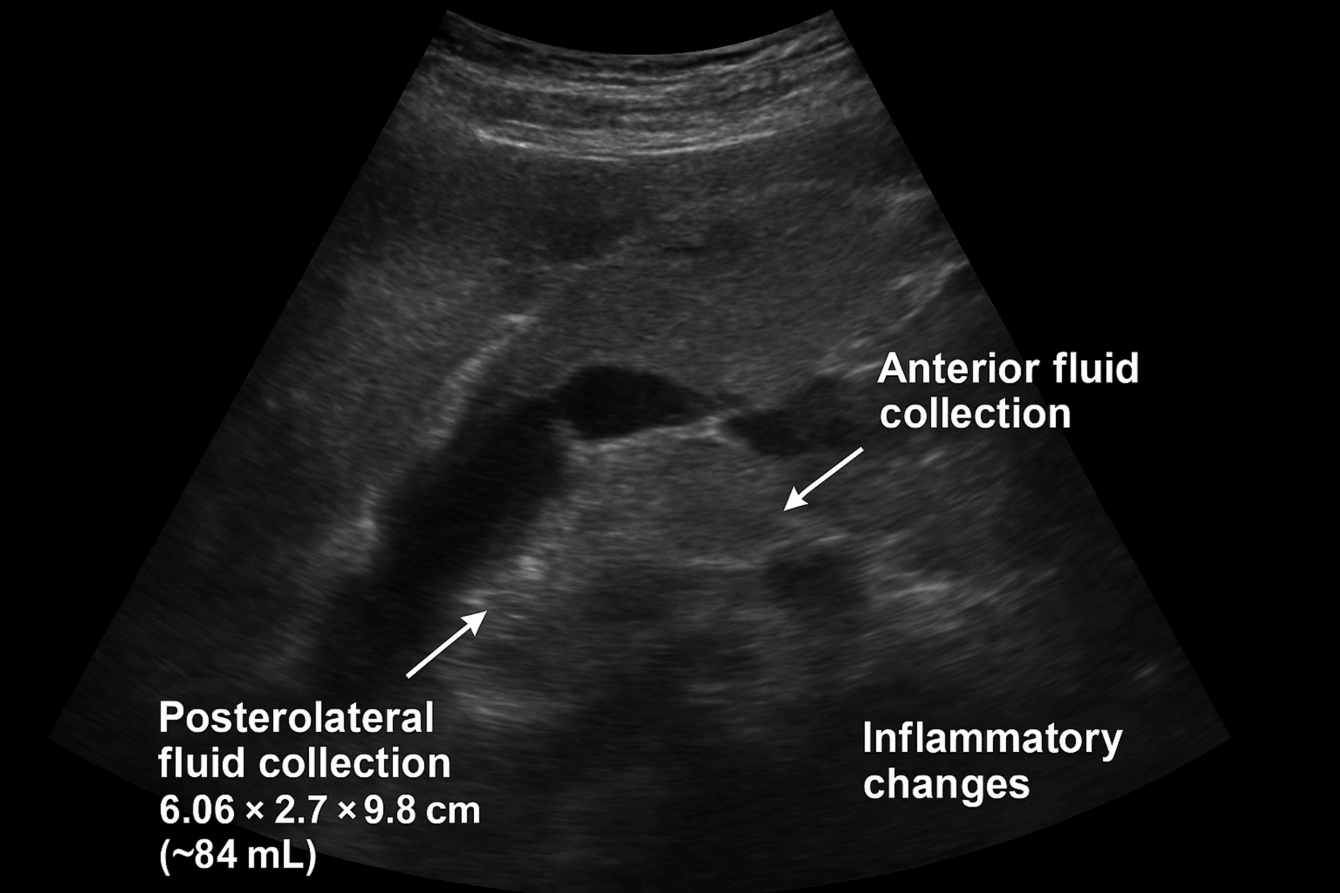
Abdominal ultrasound showing inflammatory changes in pancreatic parenchyma with fluid collections (Nov 04, 2024)
